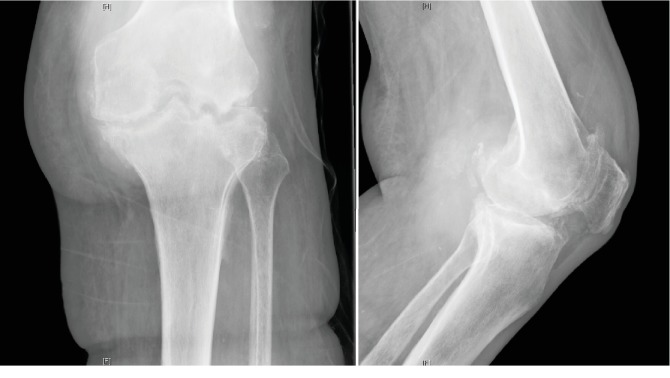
Left knee anteroposterior (AP) and lateral plain films demonstrating joint space narrowing with erosive features.
